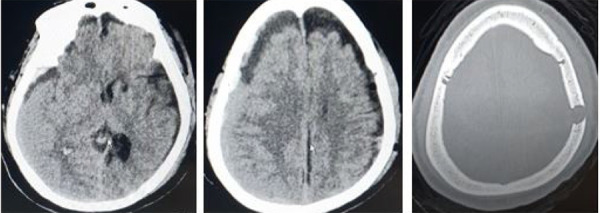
Postoperative follow up head CT showing expansion of unilateral hematoma and slight midline shift.
